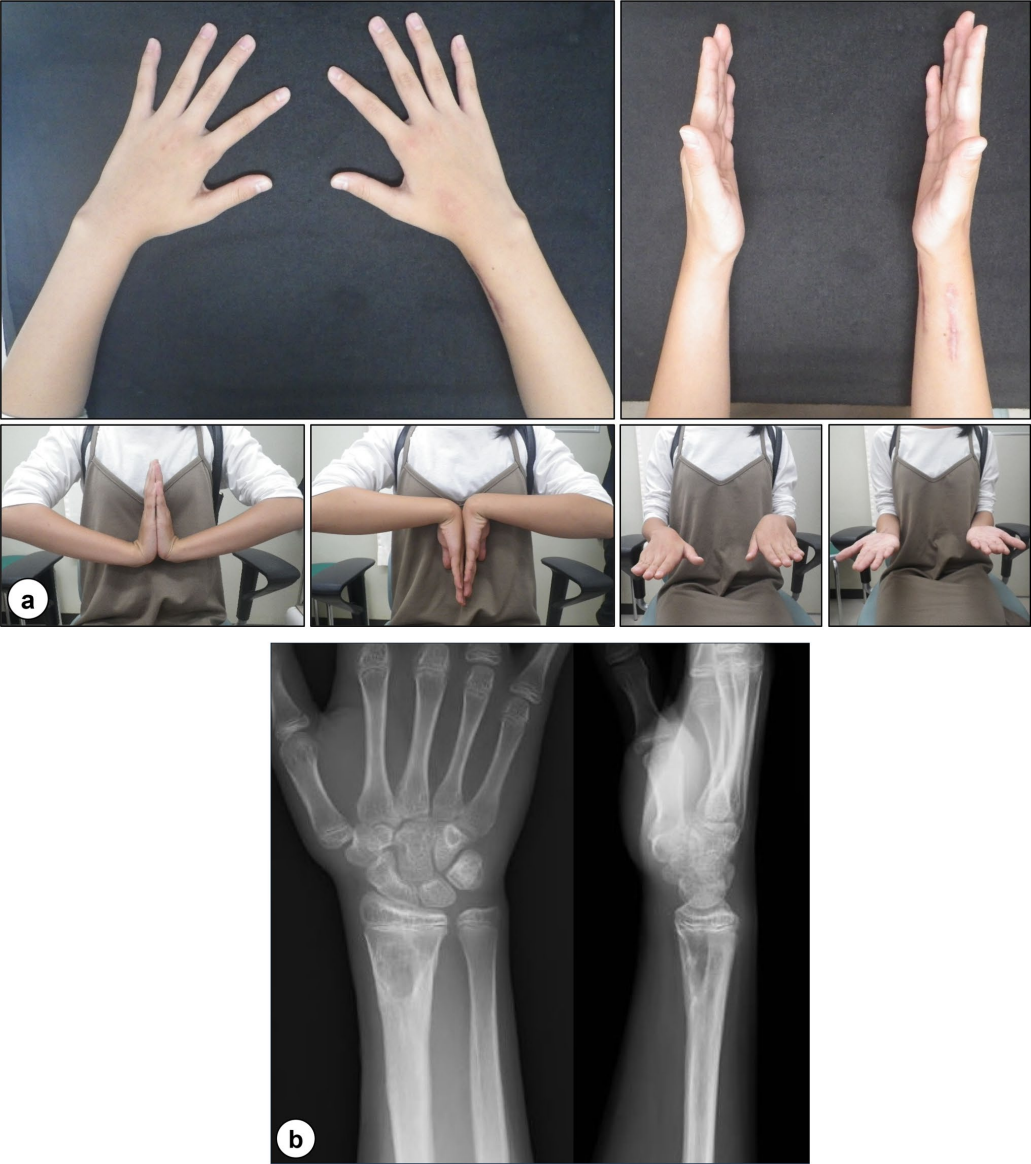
Postoperative radiographs: a photographs and b anteroposterior (left) and lateral (right) radiographs
